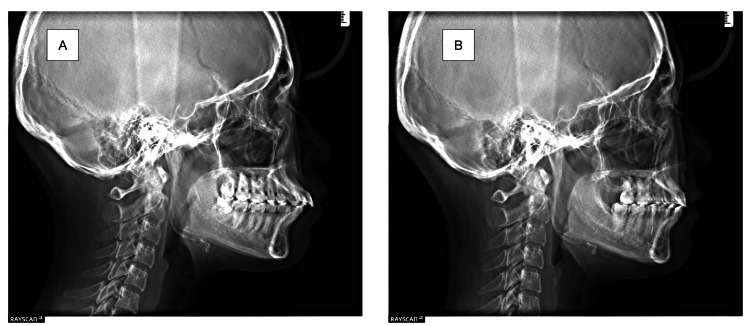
Comparison of lateral cephalometric radiographs (A) Preoperative lateral cephalometric radiograph. (B) Postoperative lateral cephalometric radiograph.
